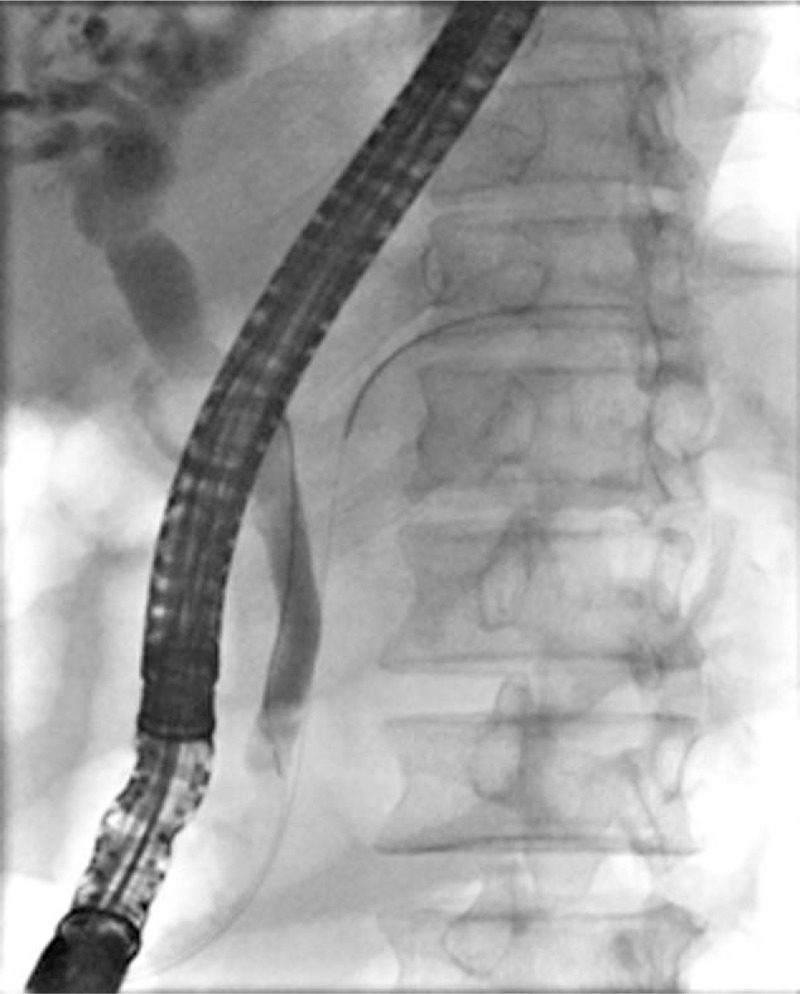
Radiograph showing an 18-mm gallstone compressing onto the CHD at the level of the cystic duct, with its upstream CHD and intrahepatic bile ducts dilation. CHD = common hepatic duct.
